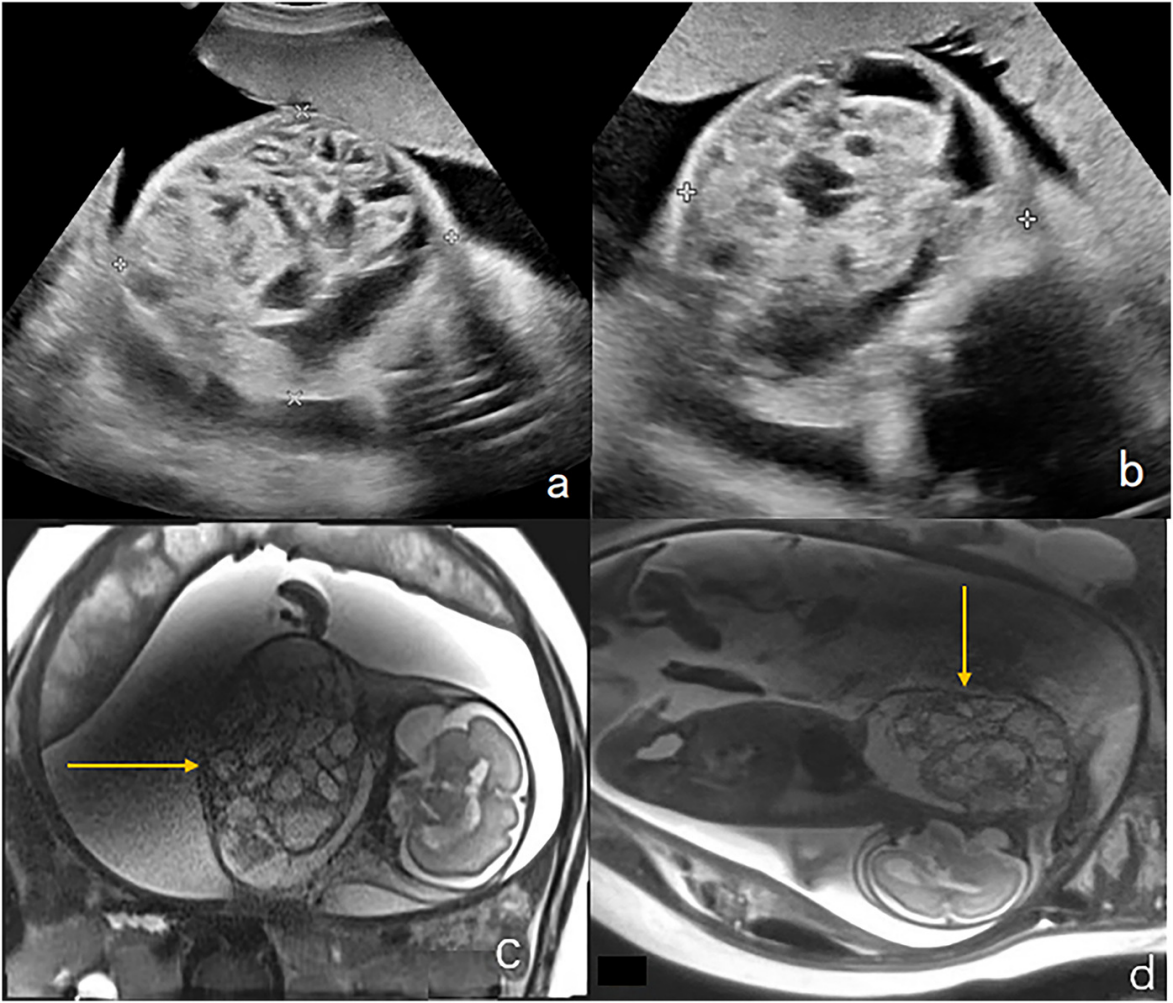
Immature teratoma in the anterior neck of a fetus (case 17). (a,b) Ultrasound examination at 23 weeks of gestation revealed a mass in the front of the neck measuring 8.6 × 7.2 × 6.8 cm, with a maximum amniotic fluid depth of 7 cm. (c) Coronal T2-weighted magnetic resonance imaging showed an irregular solid cystic mass in the front neck of the fetus, with multiple compartments, long T2 signals, and solid T1 and T2 soft tissue components(shown by the arrows). (d) Sagittal T2-weighted magnetic resonance imaging, the fetal head was substantially tilted posteriorly. The mass and amniotic fluid increased gradually over the following weeks. The maximum mass diameter and amniotic fluid depth were 14 cm and 13 cm, respectively, at 31 weeks of gestation. An emergency cesarean section was performed at 31 weeks + 5 days of gestation due to premature membrane rupture. Intubation during ex utero intrapartum treatment failed and the newborn died of asphyxia. The postoperative pathology showed that the mass was an immature teratoma of the neck.
